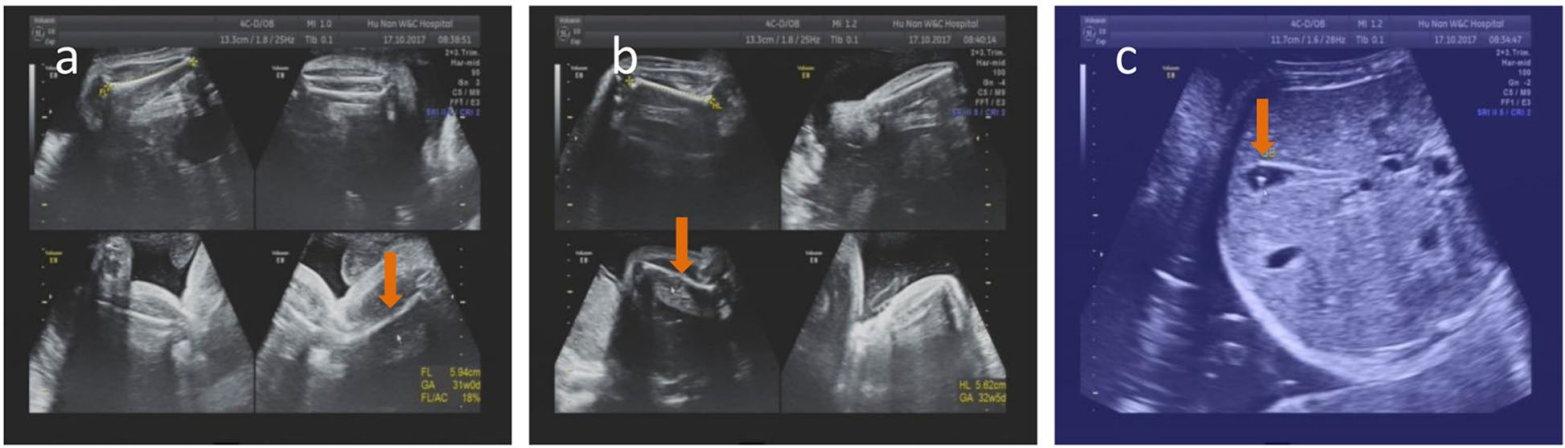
Ultrasound features of the fetus in patient 2. Short femurs (a) and humeri (b) and high echo spot in gallbladder crystals (c) are indicated with red arrows
